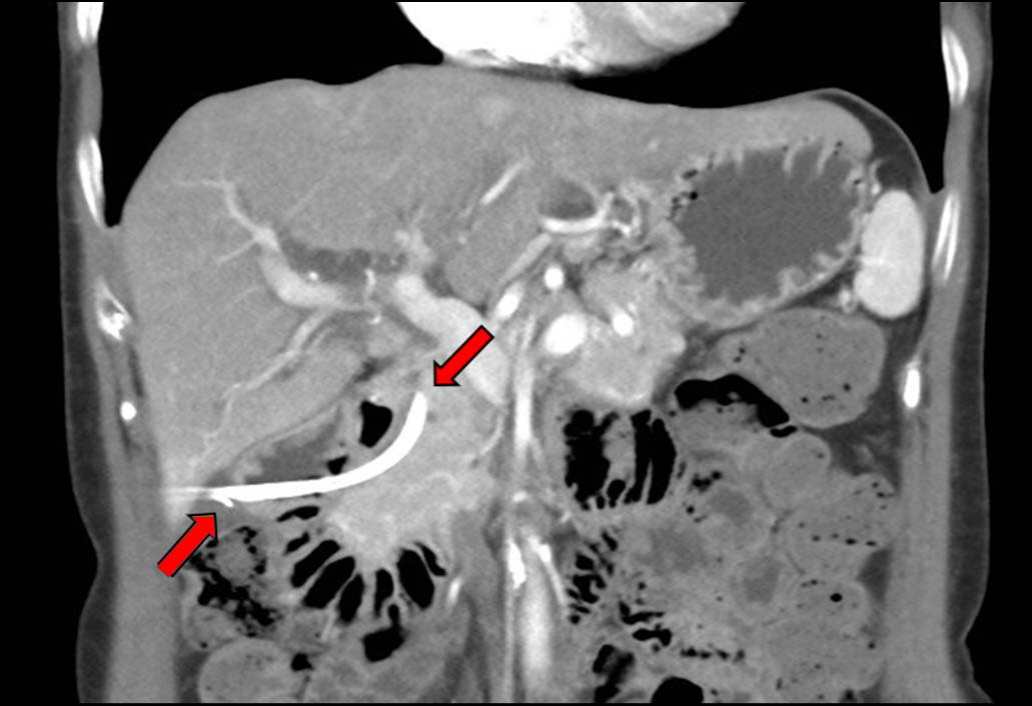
Abdominal computed tomography scan showing duodenal perforation from the distal tip of the biliary stent, whereas its proximal aspect is still within the common bile duct. Red arrows indicate proximal and distal ends of the stent.
